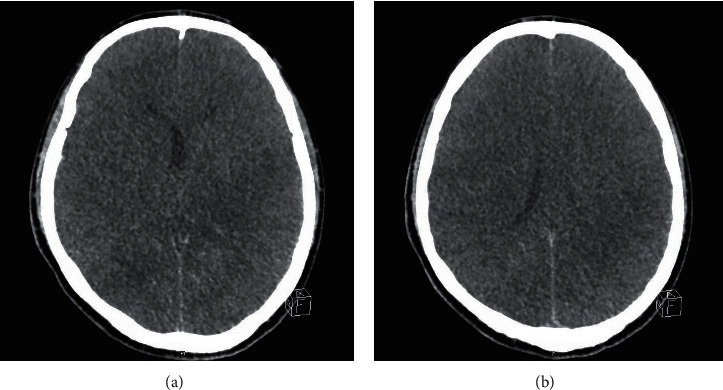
CT head slides showing brain edema on the 6^th^ day of admission.
